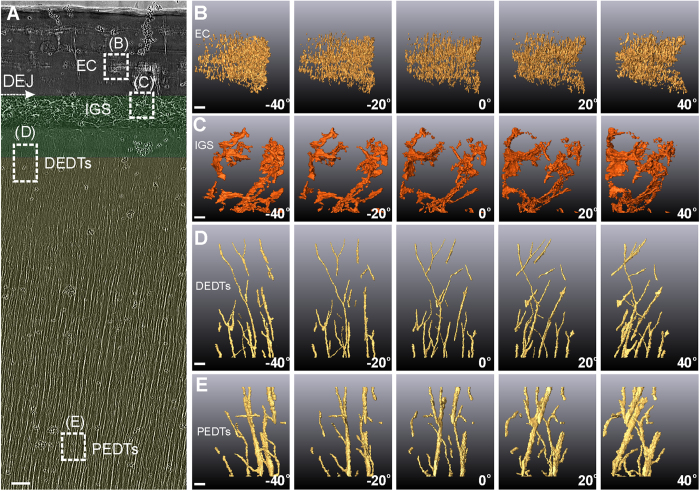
Ultrahigh-resolution internal microstructures of a *Tarbosaurus* tooth obtained via TXM. (A) 2D X-ray photograph of a sectioned *Tarbosaurus* tooth. Mantle dentin and dentin are colored translucent green and yellow, respectively. Enamel is uncolored. (Scale bar: 10 μm.) White-dashed rectangles indicate the 3D reconstruction regions shown in (B–E): (B) enamel crack (EC); (C) interglobular porous space (IGS) structures; (D) distal ends of the dentinal tubules (DEDTs); and (E) proximal ends of the dentinal tubules (PEDTs), which are approximately 190 μm away from the DEJ. (Scale bars: 1 μm.) (The small black dots in (A) are gold particles, which are used for system and image positioning).
